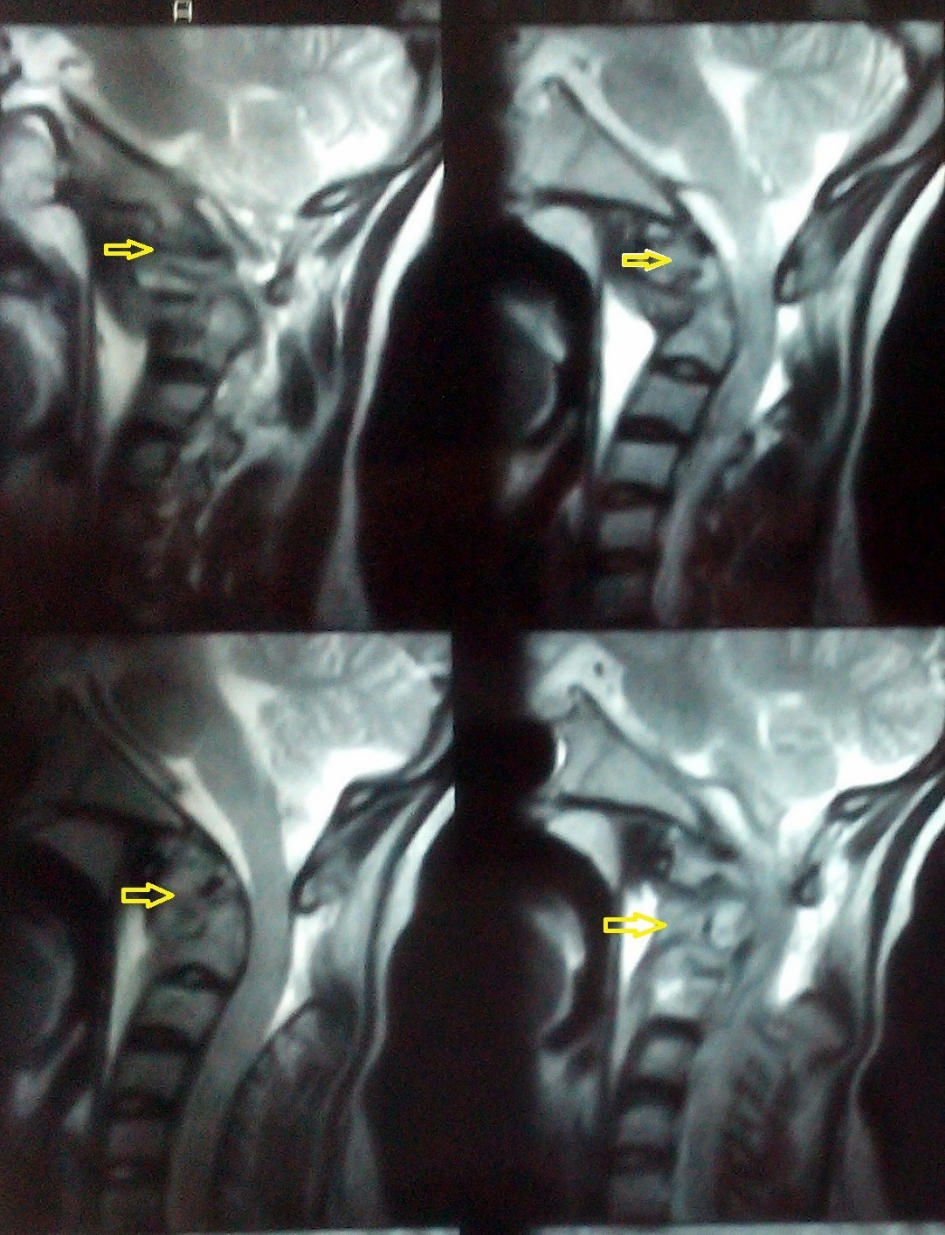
MRI of the Cervical Spine T2 weighted images showing lesions in the C1, C2, and C3 vertebrae (arrows) with resorption of the odontoid process.
